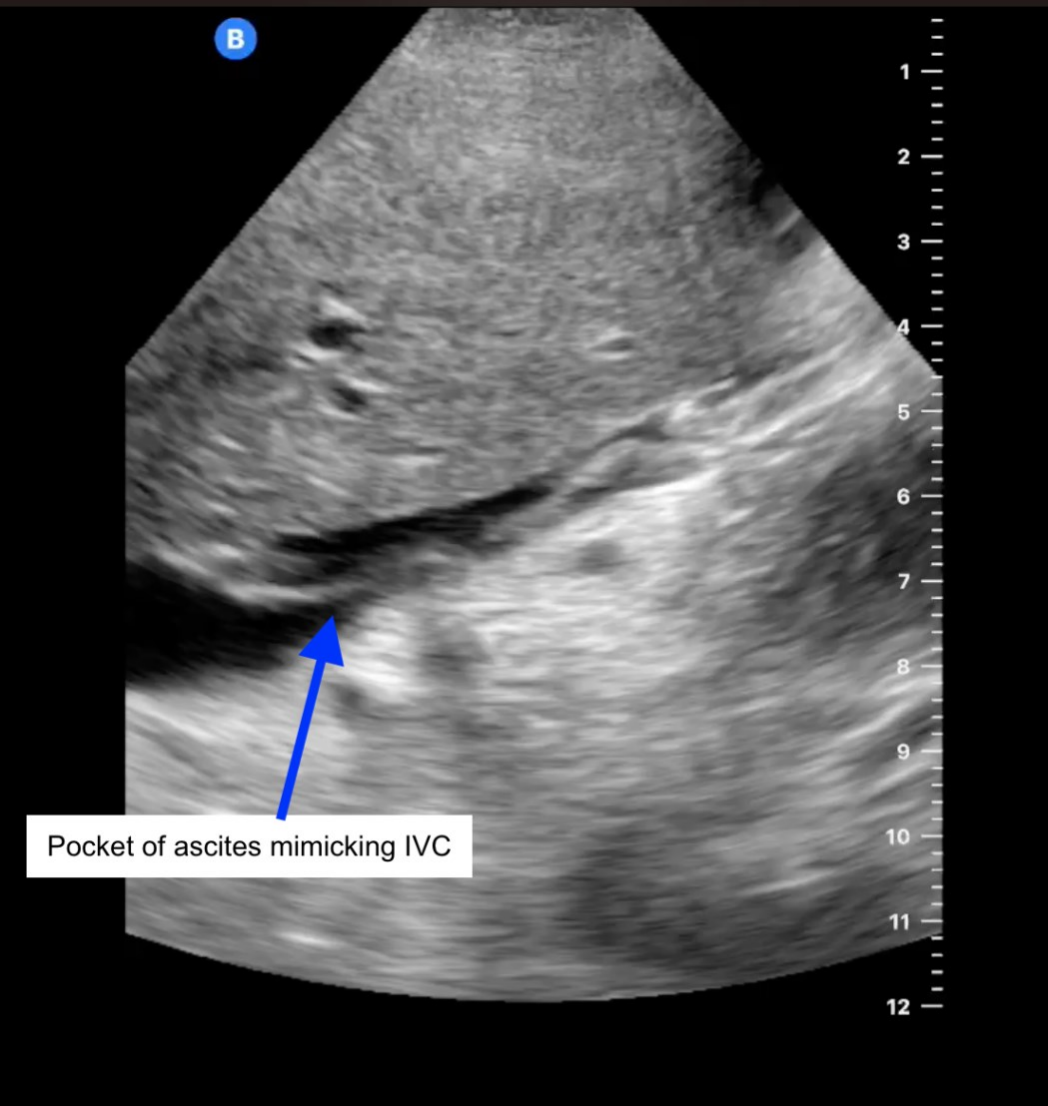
Ascites pocket with stranding – initial mimic for IVC raising concern for IVC thrombus. Color Doppler ruled out presence of vascular structure. (POCUS subcostal view in longitudinal axis. Probe is in subcostal position with marker pointing cranially).
